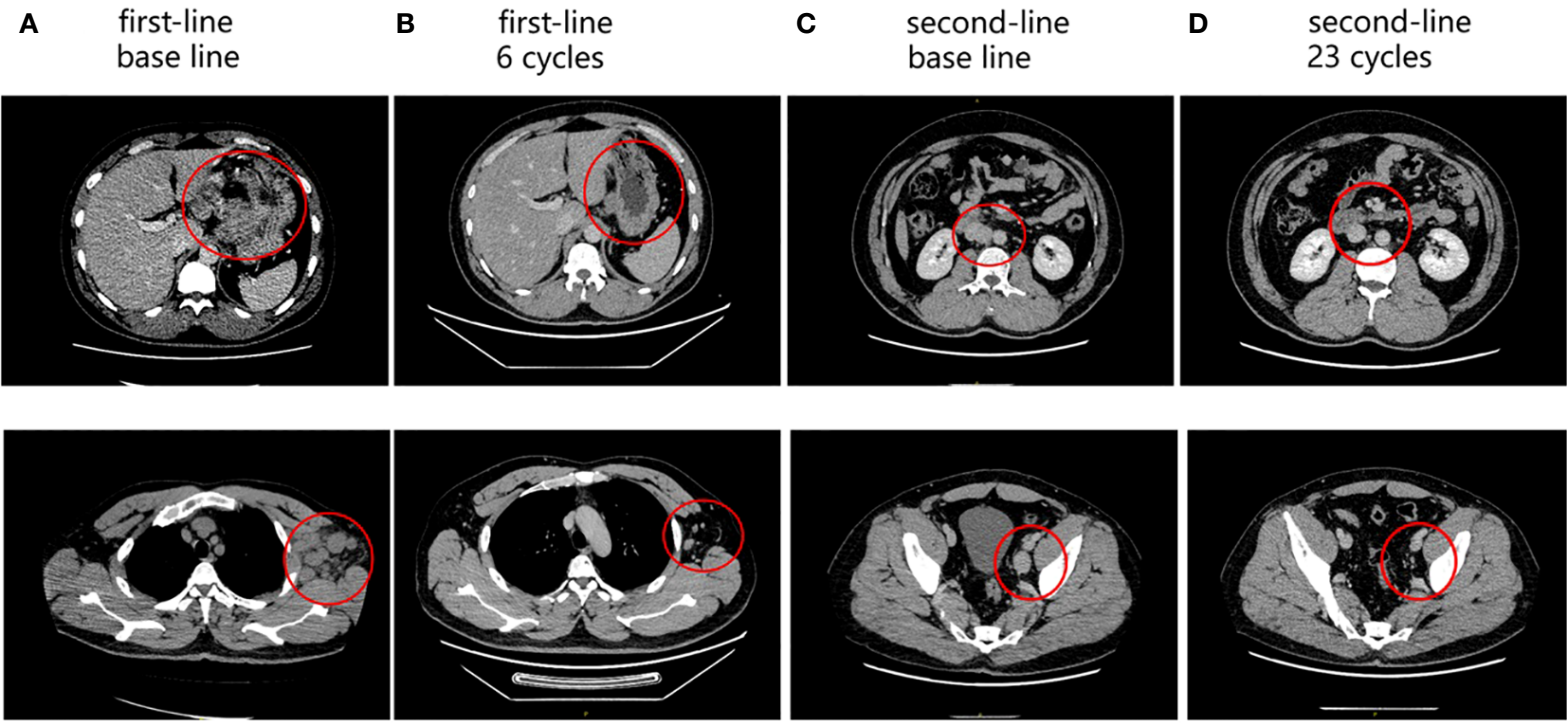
Enhanced abdominal CT during the follow-up period. (A) Baseline of first-line treatment: perigastric LNs (up); axillary LNs (down). (B) After six cycles of first-line treatment: perigastric LNs (up); axillary LNs (down). (C) Baseline of second-line treatment: retroperitoneal LNs (up); pelvic LNs (down). (D) After 23 cycles of second-line treatment: retroperitoneal LNs (up); pelvic LNs (down). The red circles represent the target lesion.
